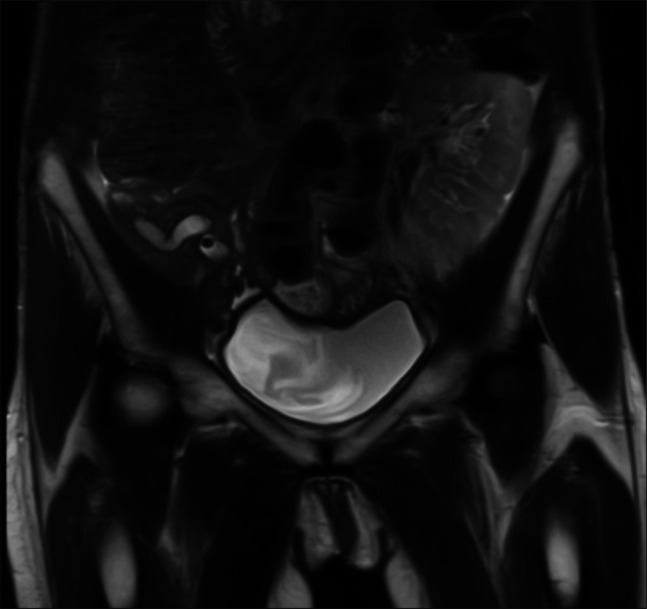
MRCP coronal T2-weighted sequence demonstrates the right lower quadrant transplant allograft and dilation of the main pancreatic duct to 1.0 cm containing nonobstructing stones. MRCP, magnetic resonance cholangiopancreatography.
